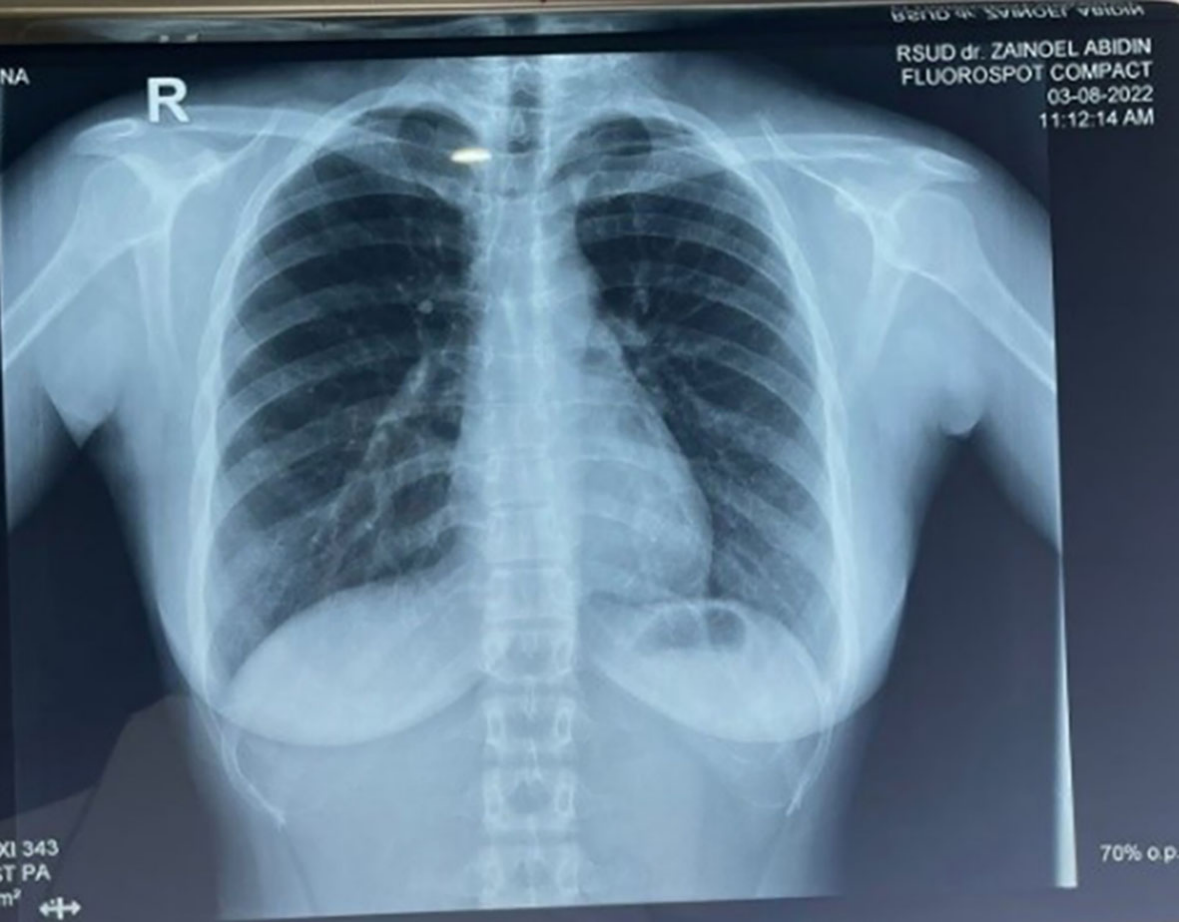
Chest radiograph PA view.
